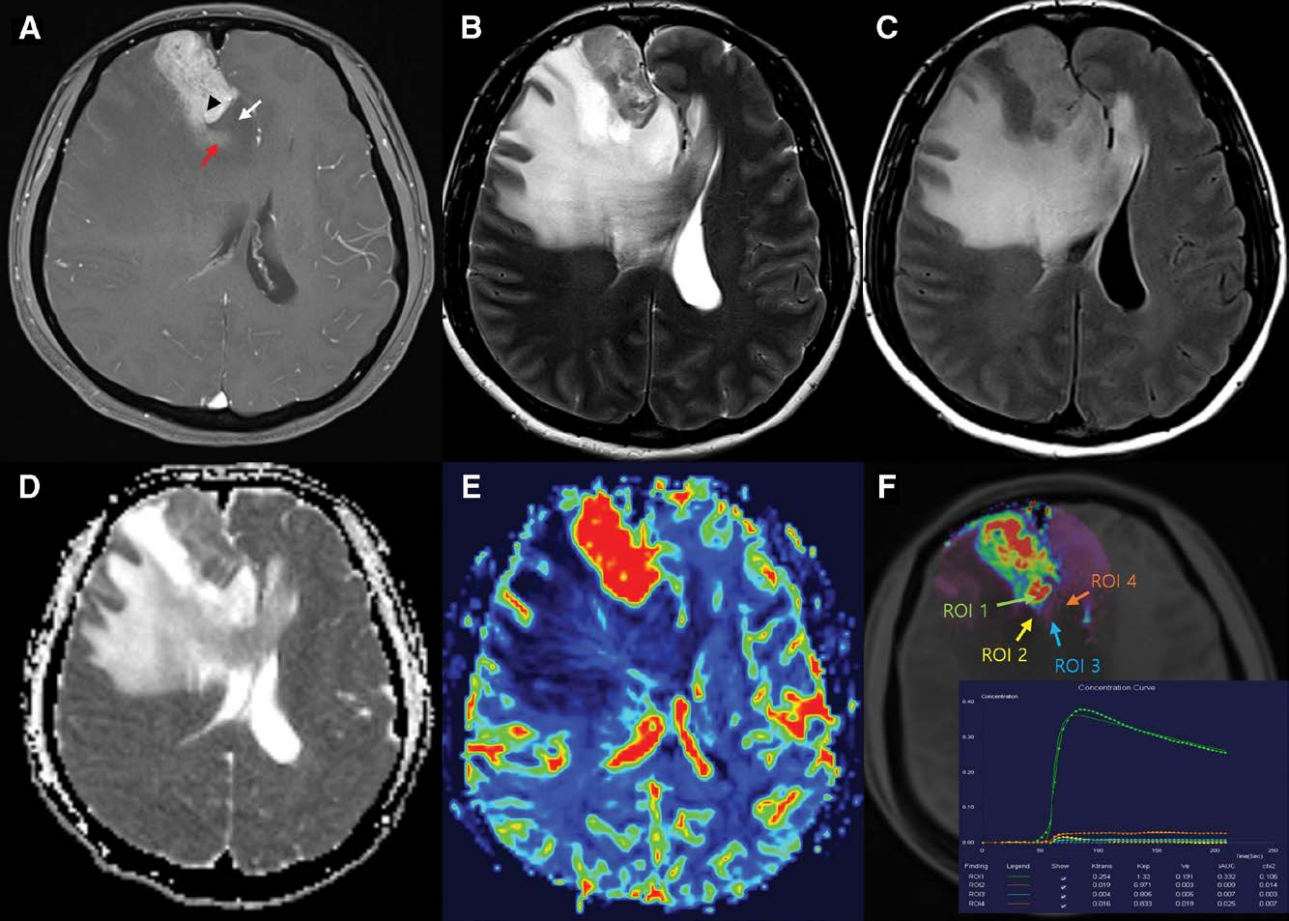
Case 1: radiologic findings of a metastatic brain tumor with contrast media leakage. (A) Contrast-enhanced brain magnetic resonance imaging (MRI) reveals a 4-cm heterogeneously-enhancing mass with contrast media leakage on the right frontal lobe (tumor: arrowhead, contrast media leakage within edema: red arrow, and non-contrast leakage within edema: white arrow). The tumor has iso-to-high intensity signals, and contrast leakage has high-intensity signals similar to edema on (B) T2-weighted MRI and (C) fluid-attenuated inversion recovery (FLAIR). Contrast leakage showed higher values and was distinct from the tumor margin on (D) apparent diffusion coefficient (ADC) maps. (E) Dynamic susceptibility contrast (DSC) and (F) dynamic contrast-enhanced (DCE) reveal the tumor has increased cerebral blood volume (CBV) and a high K trans value and contrast leakage with a low CBV and low K trans value [tumor: region of interest (ROI) 1, contrast media leakage within edema: ROI 2, 3, non-contrast media leakage within edema: ROI 4].
